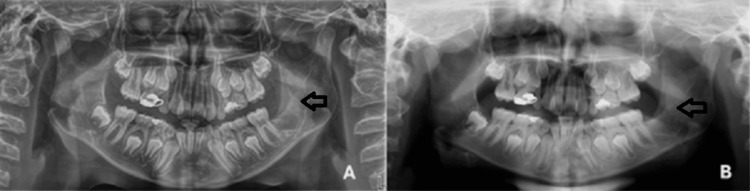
Postoperative orthopantomograms of the patient (A) one-month postoperatively and (B) at the one-year follow-up examination demonstrating significant bone remodeling of the defect, without evidence of recurrence.
